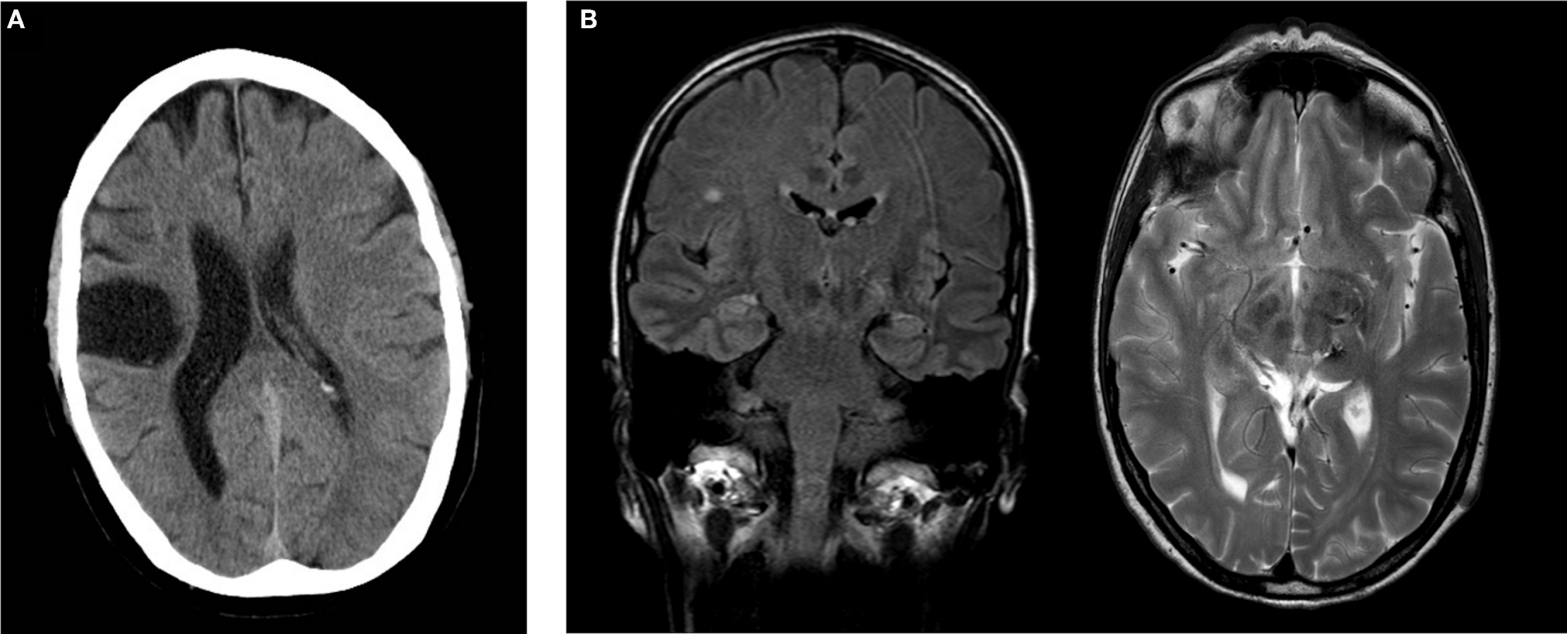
(A) Postischemic cranial CT of subject AA showing extensive postischemic necrosis in the pericentral right MCA distribution. (B) Postoperative cranial MRI of subject CC demonstrating a focus of gliosis in the left thalamus with discreet hemorrhagic residua on a T2w image. Incidental finding of single subcortical microangiopathic gliosis in the right centrum semiovale on FLAIR image. Coronal FLAIR image (left), transverse T2w image (right).
